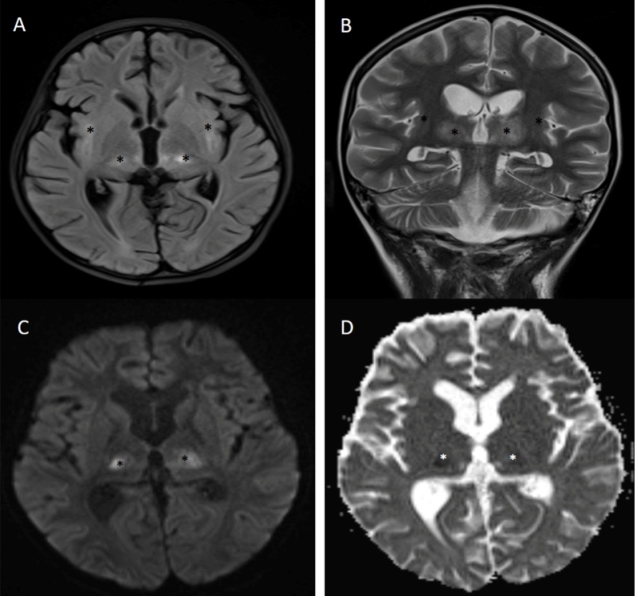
(A) Axial FLAIR image and (B) coronal T2-weighted image show the decreased symmetrical hyperintense lesions in the bilateral thalami and external capsules (black asterisks). (C) Axial b-1000 image (black asterisk) and (D), axial ADC diffusion image (white asterisk) show the regression of symmetrical diffusion restriction pattern in the bilateral thalami
